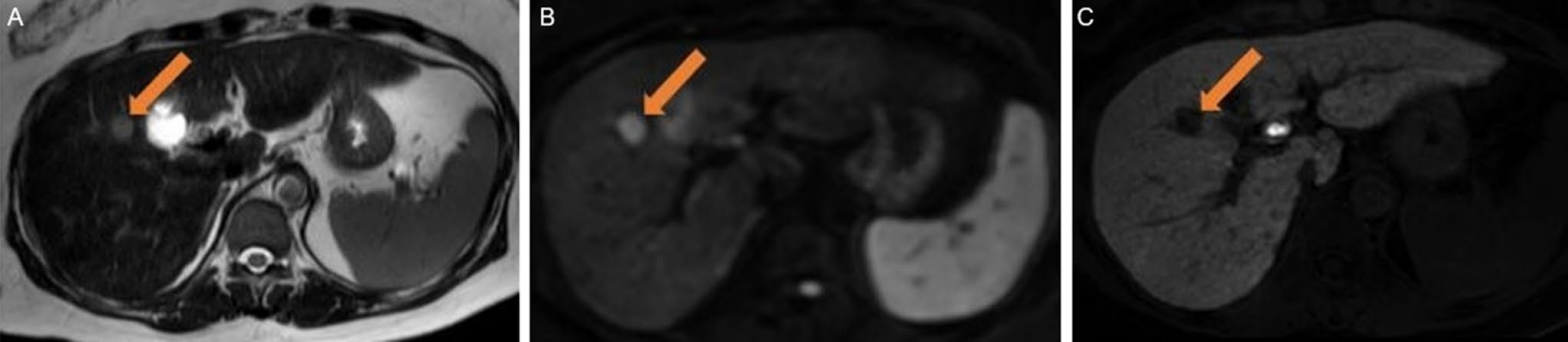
Positive surveillance HBP-AMRI. Axial T2-weighted (A) MR image shows a small mildly T2 hyperintense mass adjacent to the gallbladder fossa (arrow). (B, C) Axial diffusion-weighted and contrast-enhanced hepatobiliary phase MR images show that the mass has restricted diffusion and is hypointense to background liver parenchyma on hepatobiliary phase indicating the presence of non-functioning hepatocytes. HCC was confirmed on full diagnostic MRI with an extracellular contrast agent
